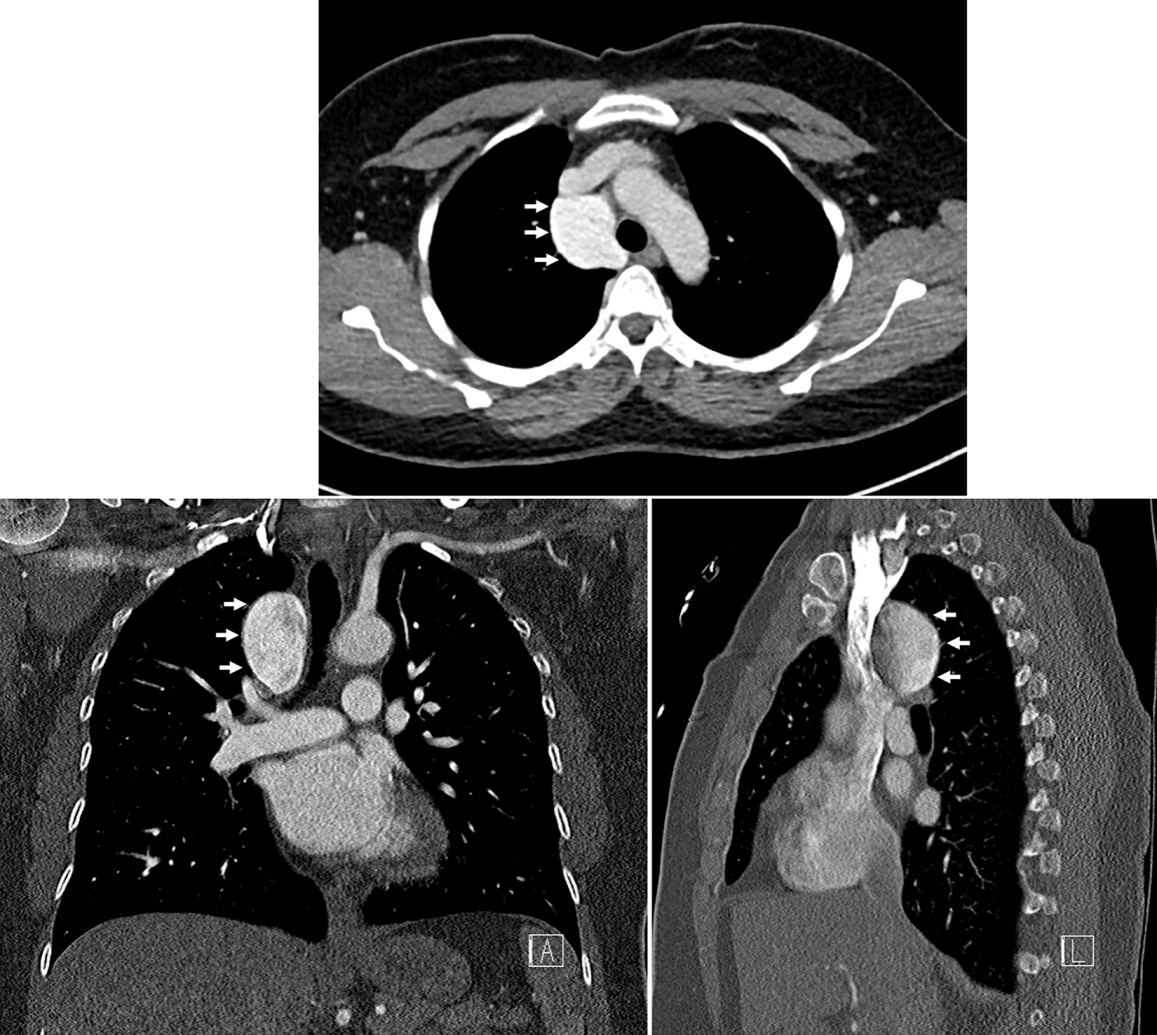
Chest computed tomography demonstrating a 4.4 × 4.1 × 3.6 cm mediastinal lesion adjacent to the superior vena cava and ascending aorta (white arrows), exhibiting contrast density with the appearance of flow but without direct communication to other vascular structures.
